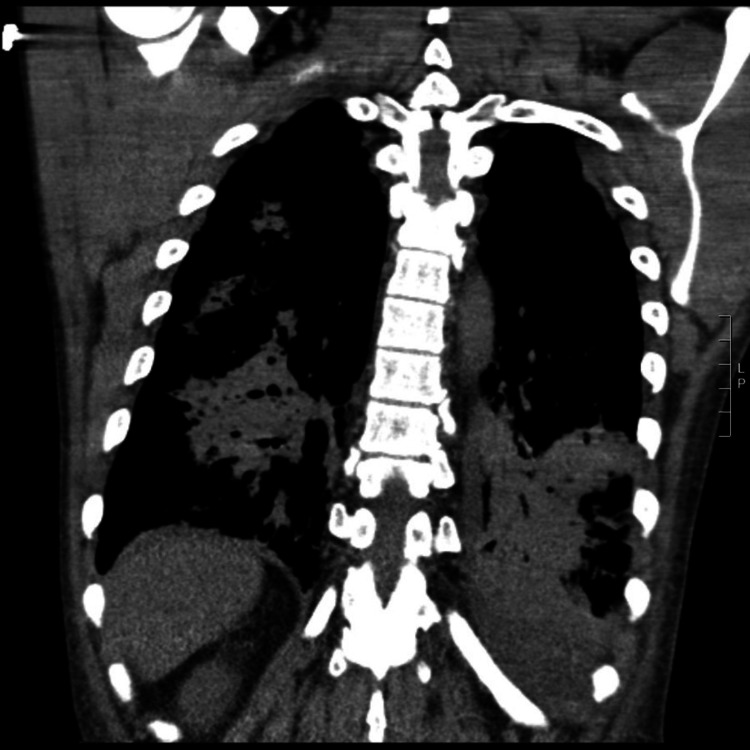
Computed Tomography (CT) of the Chest CT of the chest shows bilateral consolidations, concerning for bilateral multifocal pneumonia secondary to human metapneumovirus.
